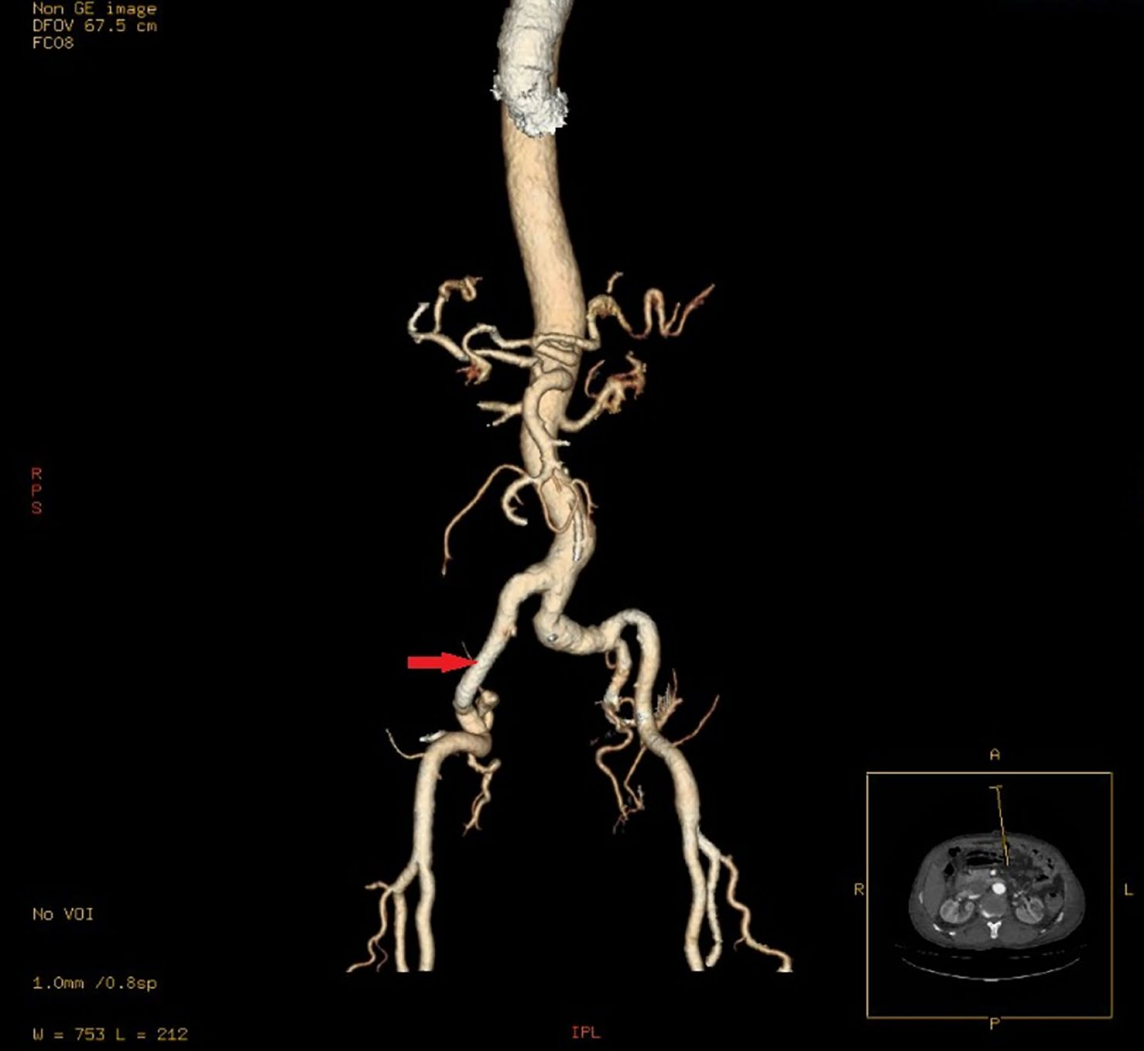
3D CT scan view post repair with a Dacron graft, with the red arrow indicating the graft.
